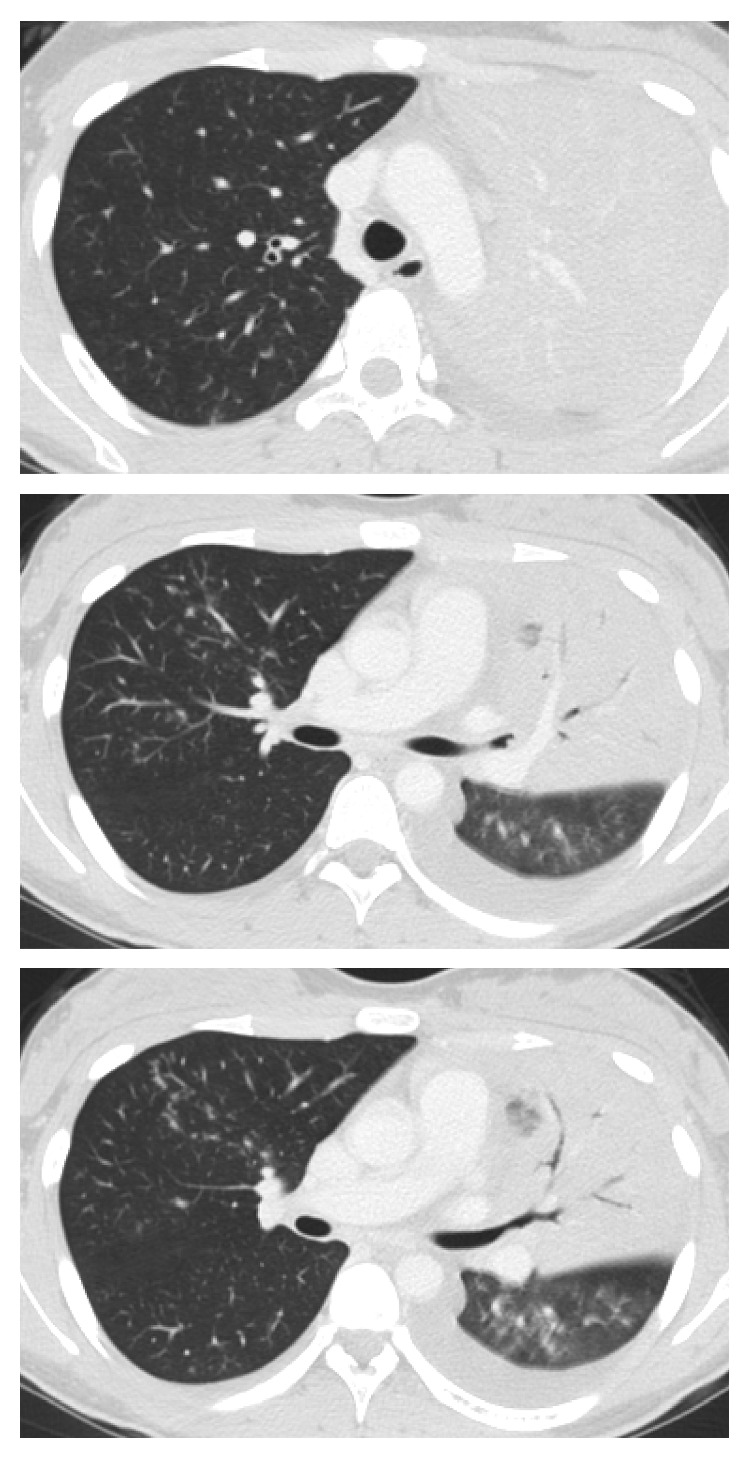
Chest computed tomography image on day 5 showing extension of the consolidation areas with air bronchogram in the left upper lobe and opacities in the right lung.
